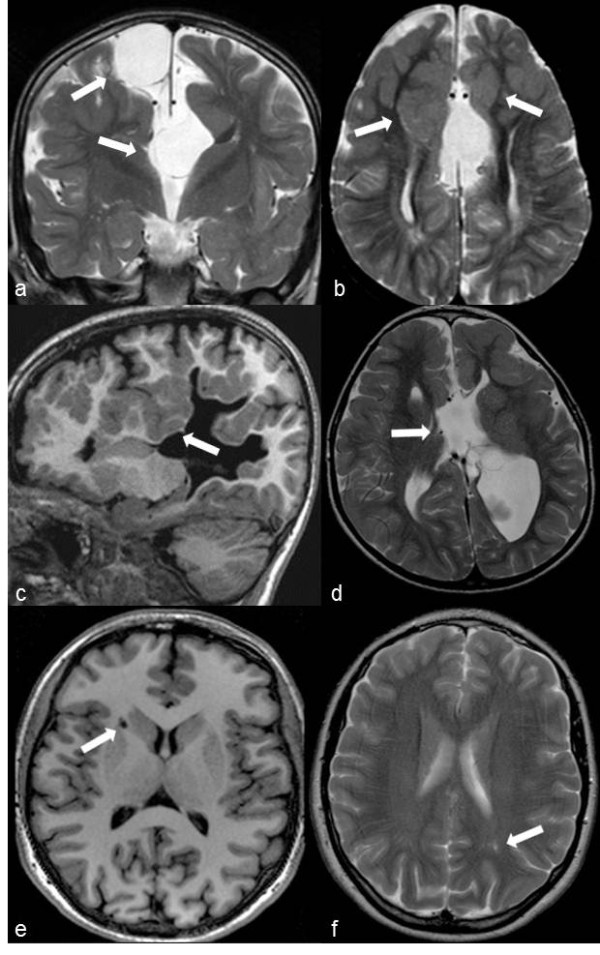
Overview of the typical neuroimaging findings in ofd1 patients. A, b: *Case ID50, 52 months* (*OFD1* mutation: 337 C > T; Q113X)*.*a) Coronal TSE T2-weighted image: corpus callosum agenesis. Two arachnoid cysts (arrows) are respectively located upper to the roof of the third ventricle and on the right side of the falx. Note the “Texas longhorn” configuration well depicted only on the left side where the Probst bundle is also well visible. b) Axial TSE T2-weighted image: thinning and parallel orientation of the lateral ventricles. Bilateral fronto-mesial subcortical heterotopias (arrows) are evident along the anterior part of the interhemispheric defect. The bigger one anteriorly truncates the right Probst bundle that is thinner than the controlateral. A subtle thickening of the frontal cortical surfaces suggestive of polymicrogyria is also evident. Note the thin multiple dilatations of Virchow-Robin spaces at the posterior white matter. c, d: *Case ID39, 8-year-old* (*OFD1* mutation: 290 A > G; E97K)*.*c) Sagittal TFE T1-weighted 3D images: note the frontal and parietal clefts surrounded by irregular and thickened grey matter consistent with infolding polymicrogyria (arrow). d) Axial TSE T2-weighted image: the interhemispheric defect is caused by the agenesis of the corpus callosum. Two arachnoid cysts are located between the outpouching of the third ventricle (arrow) and the enlarged left lateral ventricle. On the left side, polymicrogyria of the frontal cortical surface, as well as the periventricular deep portion of the infolding, are both well appreciable. e, f: *Case ID13, 13-year-old* (*OFD1* mutation: 247CAA > TAA; Q83X)*.*e, f) Axial TFE T1weighted 3D and TSE T2 images: note some mild dilatation of Virchow-Robin spaces at the supratentorial white matter (arrows).
